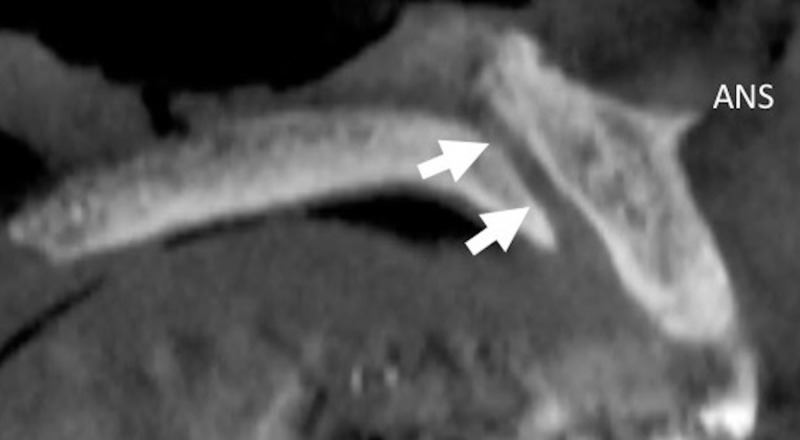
Midsagittal plane of the incisive canal (arrows) on CBCT image. ANS: anterior nasal spine; CBCT: cone-beam computed tomography
